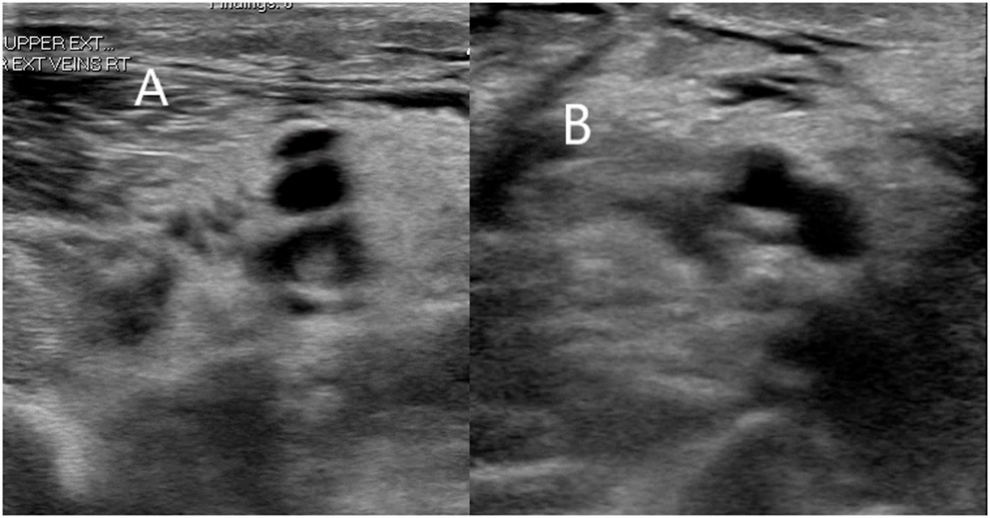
A 70-year-old man in the intensive care unit had right arm swelling after 1 week of diagnosis of COVID-19. Doppler ultrasound of the right arm showing occlusive thrombus within right brachial (A) and basilic veins (B).
